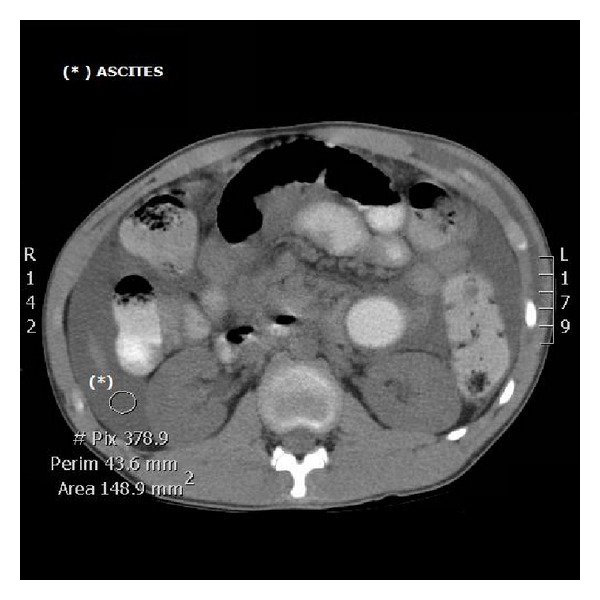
Axial CT slice demonstrating free fluid in the peritoneal cavity. The slide was made at the level of the kidneys that do not show hydronephrosis.
